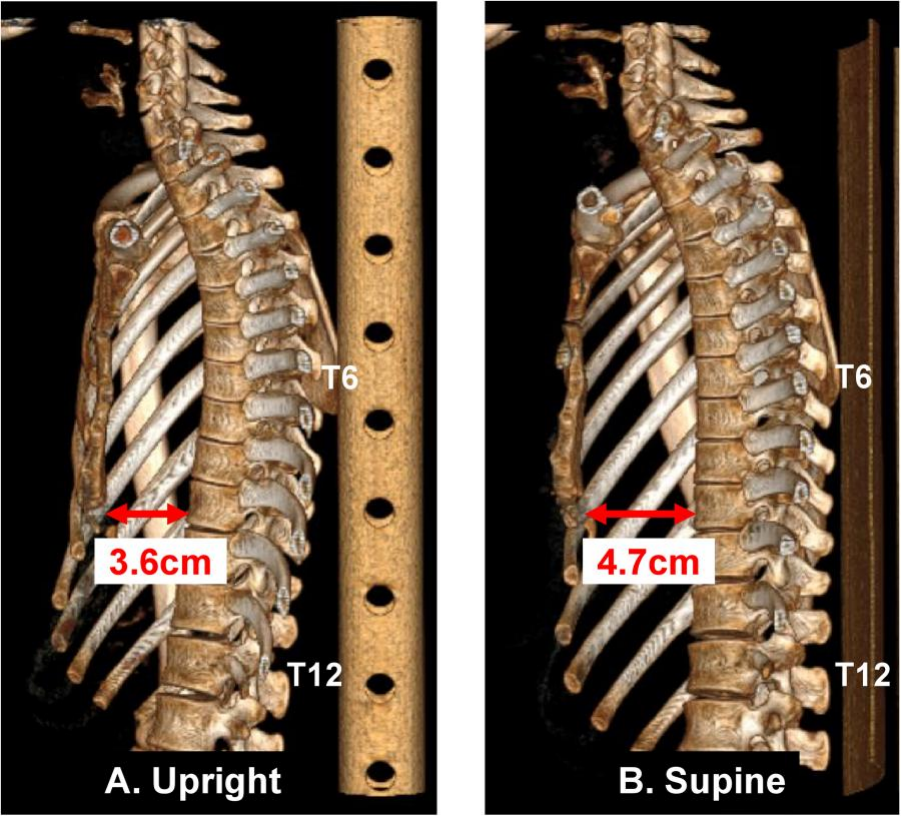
Representative sagittal computed tomography images in the upright (A) and supine (B) positions. In this patient, switching from the supine to the upright position resulted in a 1.9-cm anterior shift of the T12 plumb line relative to the T6 plumb line. The minimum anterior–posterior diameter (arrow) was shorter in the upright position than in the supine position (3.6 vs 4.7 cm).
